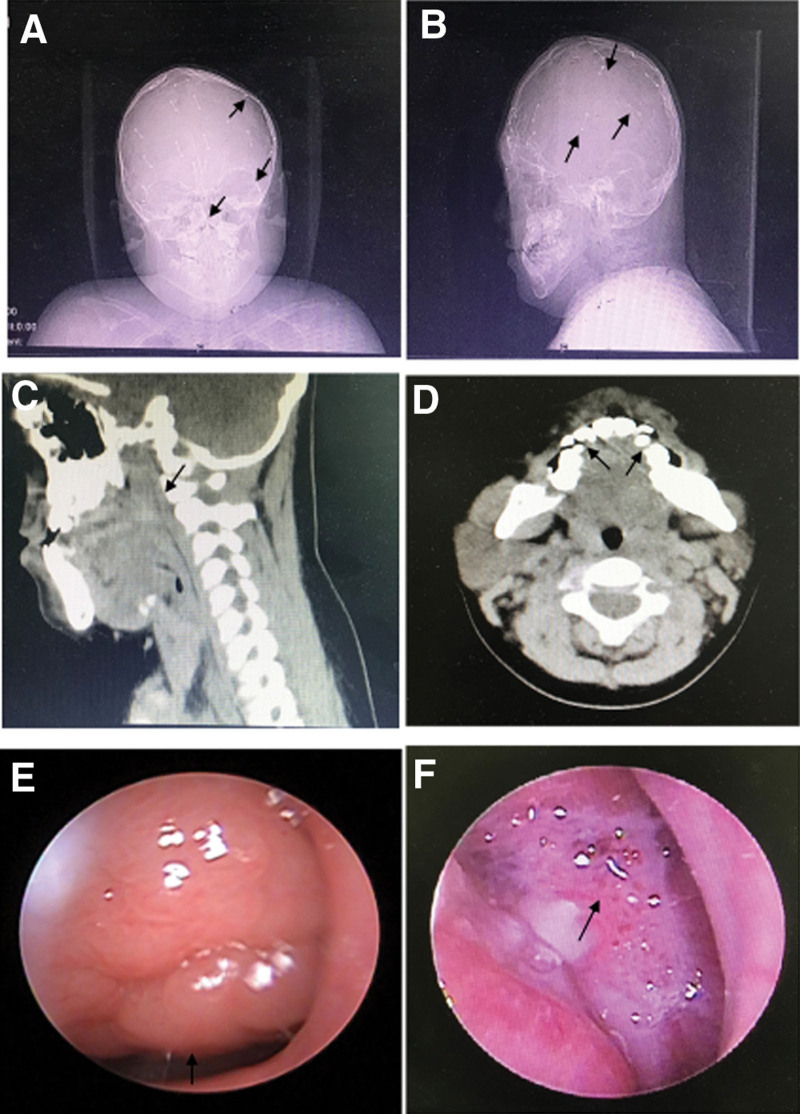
(A, B) Cranial CT showed: frontal-parietal temporal-occipital bone, bilateral orbital lateral wall and nasal bone were unnatural (shown by the arrow → in figure A), multiple metal high-density shadows (shown by the arrow → in figure B) were seen, bilateral eyeballs protruded outwards. (C) Nasopharyngeal CT showed: adenoid hypertrophy, almost completely obstructing the posterior nostril and oppressing the torus tubarius (the arrow → show hypertrophic adenoids); (D) Irregular arrangement of teeth and narrow posterior nasal meatus; (E) Nasal endoscope showed adenoid hypertrophy; (F) Reexamination of nasopharynx showed that the wound of nasopharynx recovered well.
